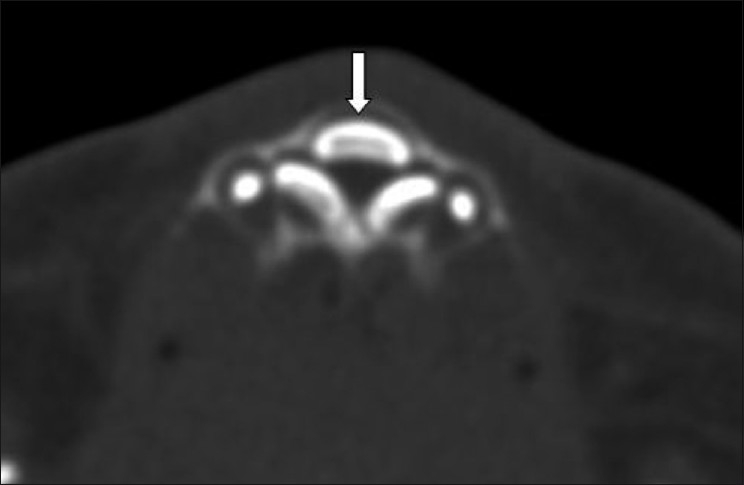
Axial CT scan shows an unerupted single central maxillary megaincisor (arrow)
